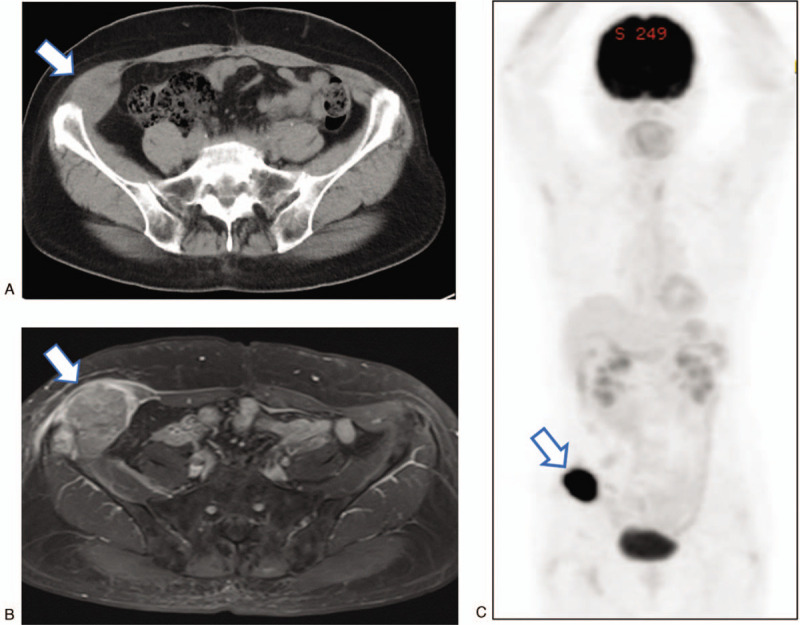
Images of the metastasis in the right oblique muscle. A, Contrast-enhanced computed tomography. B, Contrast-enhanced magnetic resonance imaging (T1-weighted). Arrows indicate a 5 x 6 cm enhancing tumor with direct involvement of the ilium. C, 2-deoxy-2-[^18^F] fluoro-D-glucose position emission tomography shows a hypermetabolic lesion in the right oblique muscle. Arrows indicate an oblique muscle tumor.
